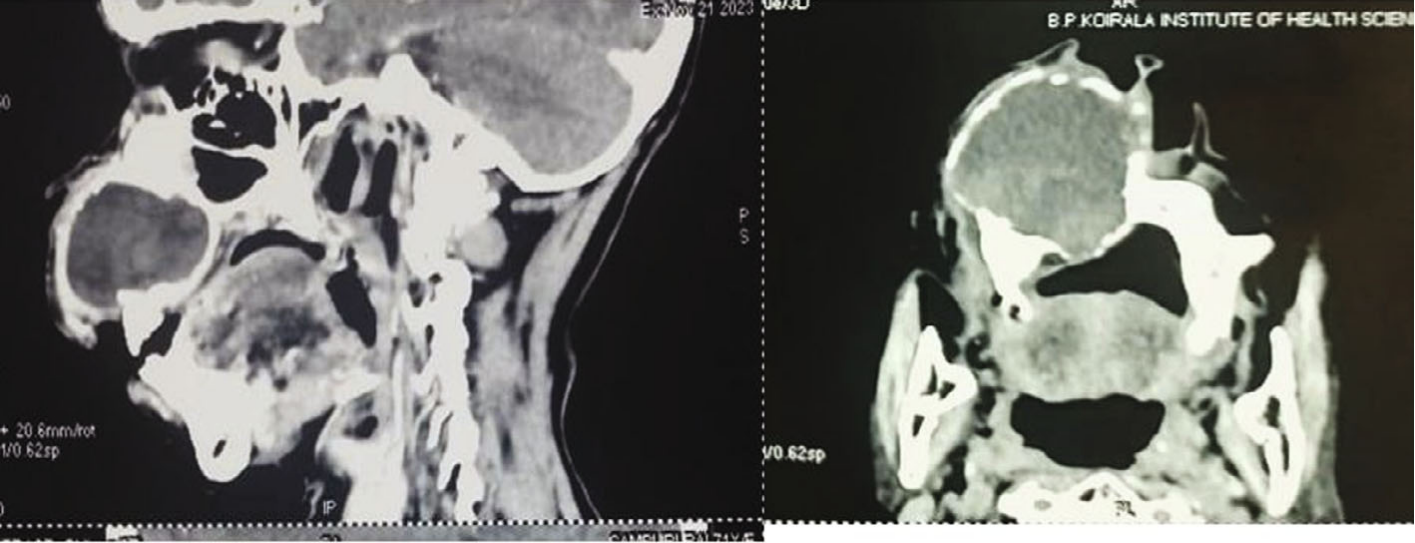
Contrast‐enhanced CT (CECT) scan (sagittal and axial sections) demonstrating an aggressive lesion with buccal alveolar expansion, cortical bone thinning, and multifocal erosion.
